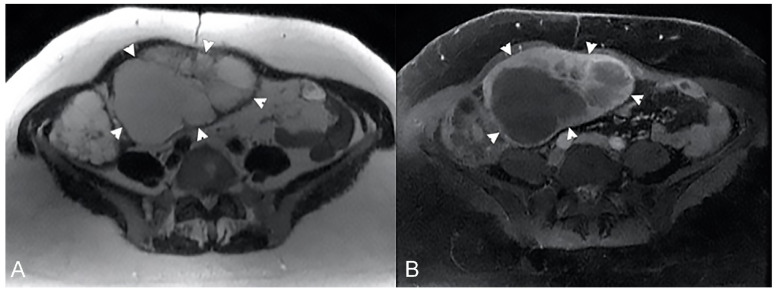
Example of marked thickening due to a malignant condition. (A) Axial T2-weighted and (B) axial T1-weighted after gadolinium injection images show a marked, focal, and asymmetrical small bowel thickening, with inhomogeneous contrast enhancement (arrowheads), caused by a malignant condition.
